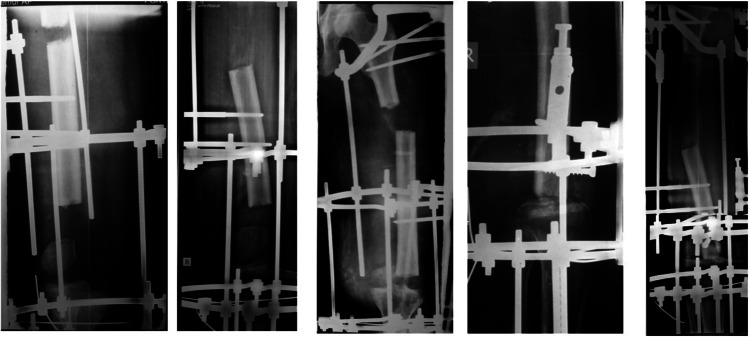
Serial X-rays of a patient during bone transport
